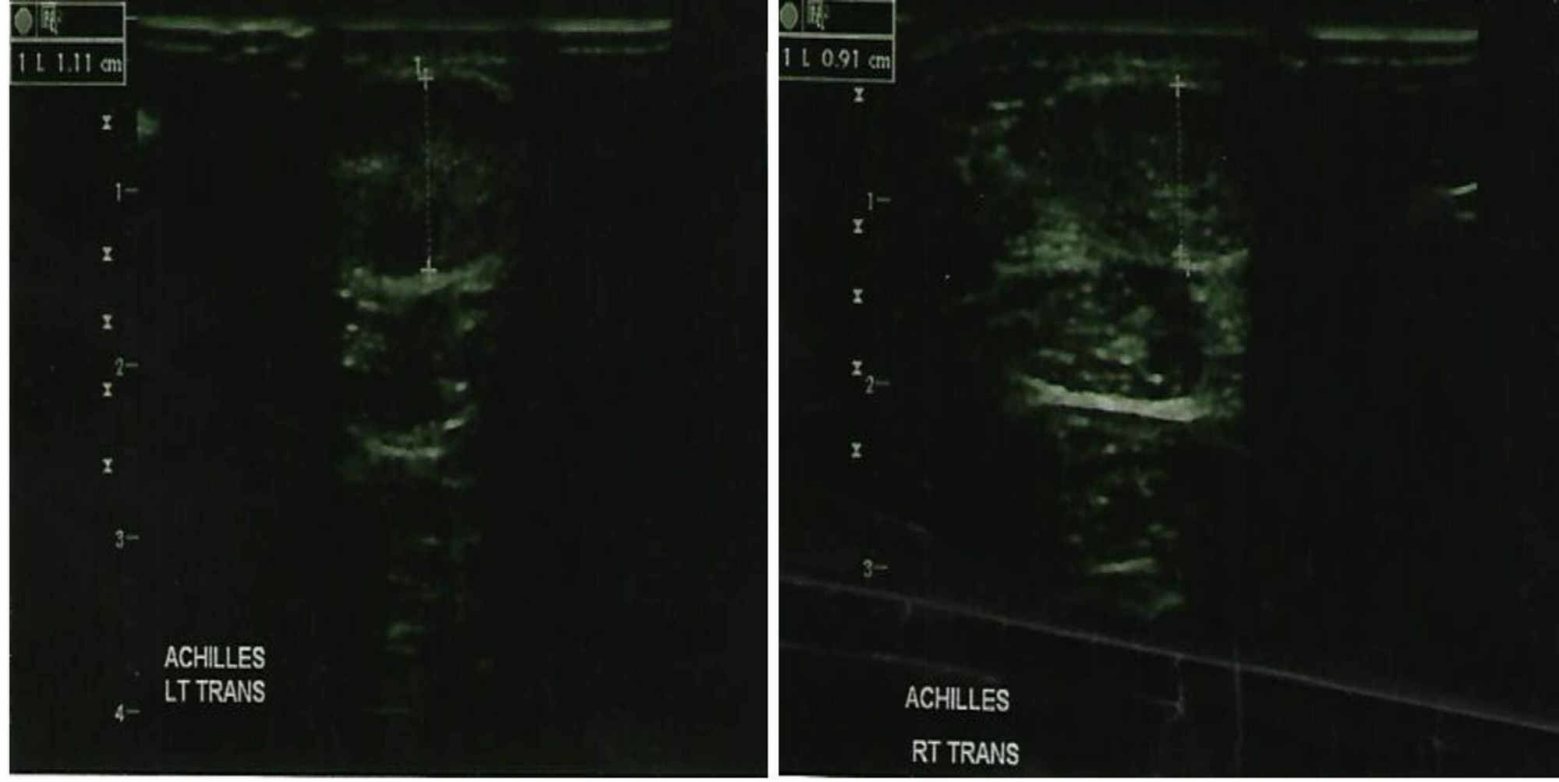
Ultrasound showing the left Achilles tendon thickness (left panel) and right Achilles tendon thickness (right panel) in 2017.
